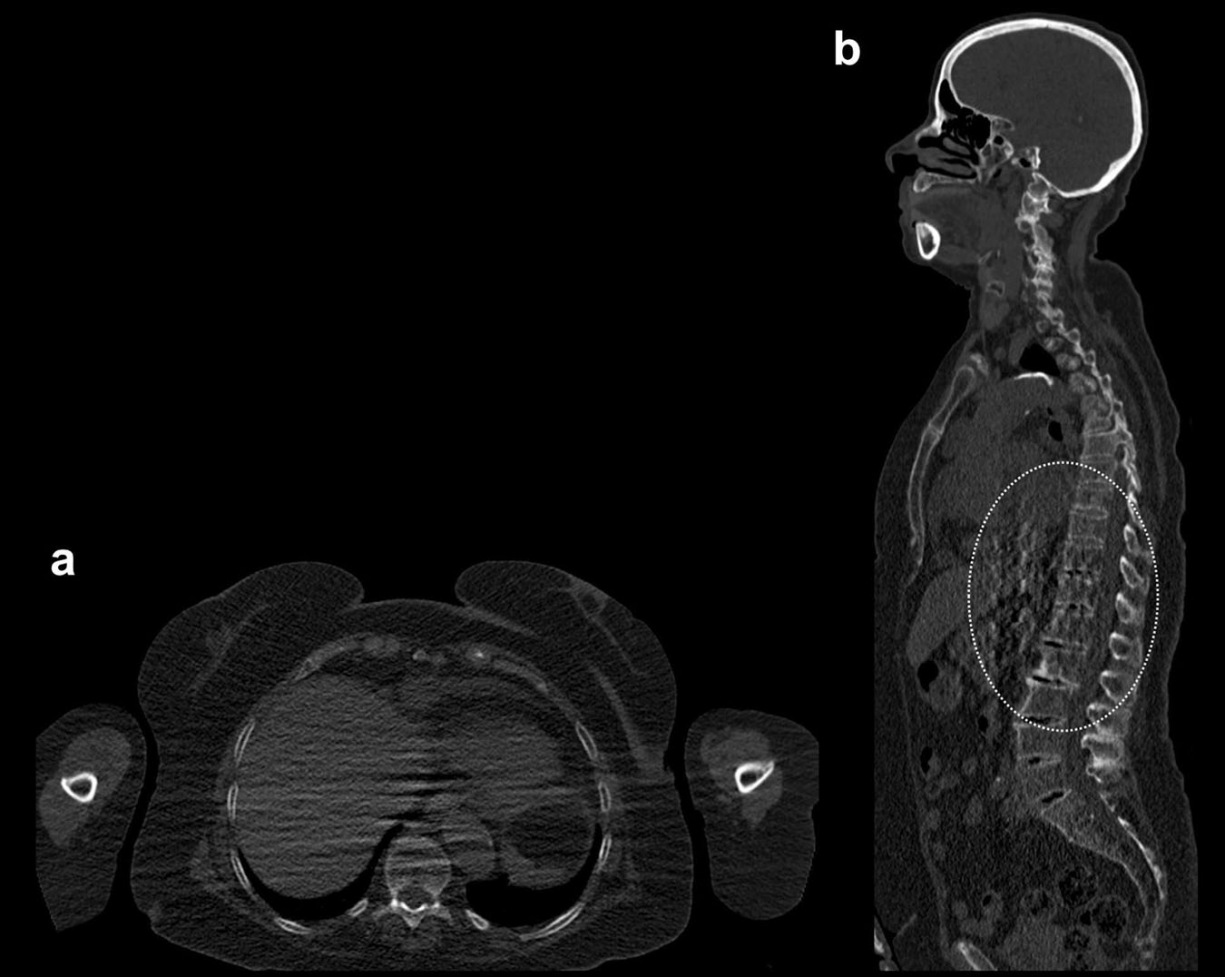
WBLDCT scan (120 kV, 60 mAs) of a 76-year-old female with newly diagnosed smoldering myeloma, performed on a 16-slice CT scanner. a Axial slice (2/1 mm thickness/increment) at the level of the T10 vertebral body. The arms are positioned alongside the body. Assessment of the vertebra is significantly impaired due to the presence of multiple superimposed streak artifacts from beam hardening. b The artifacts are also very prominent on the sagittal MPR image, mainly at the level of the lower thoracic spine (dotted ellipse). *MPR* multiplanar reformation
